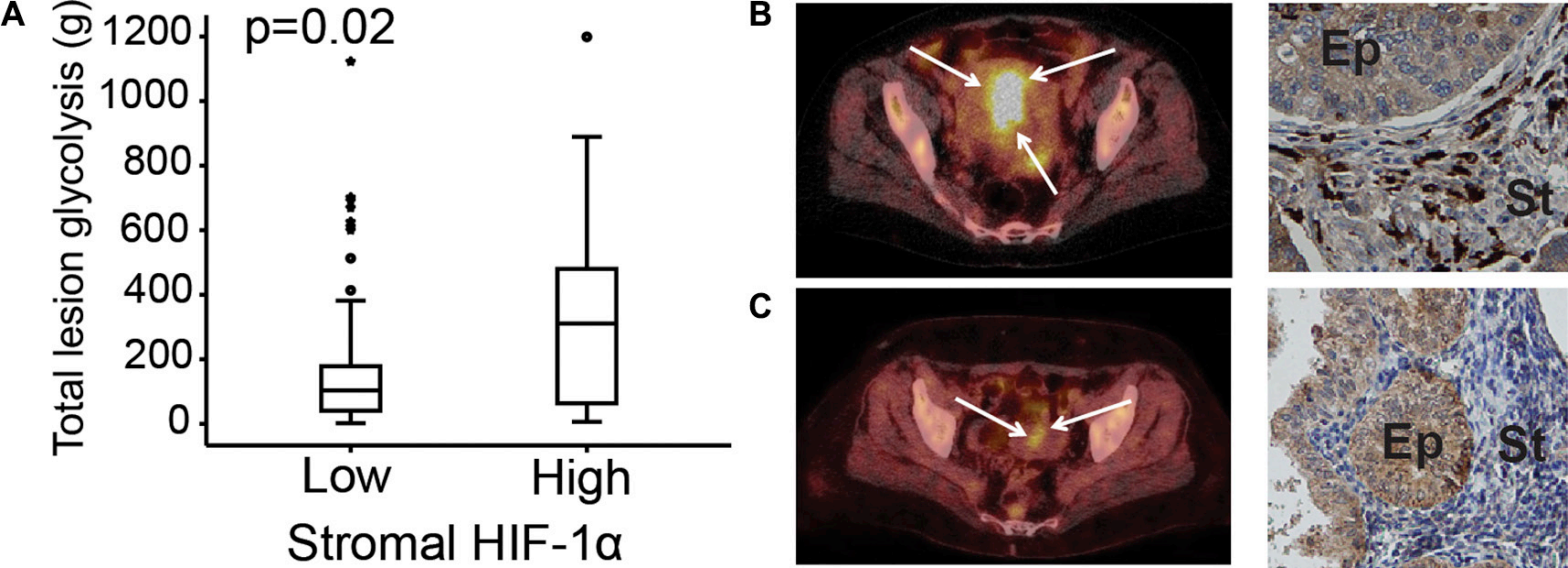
Stromal HIF-1α protein expression correlated to 18FDG PET/CT markers Total lesion glycolysis (TLG) assessed by FDG-PET/CT in primary endometrial carcinoma lesions compared to stromal HIF-1α protein expression (A). FDG-PET/CT and HIF-1α expression in a 77-year-old patient with a non-endometrioid endometrial cancer (NEEC), FIGO stage 1B: PET/CT exhibits a large FDG-avid uterine tumor (white arrows) with high TLG and the tumor had high stromal HIF-1α expression (black arrows) (B) FDG-PET/CT and HIF-1α expression in a 58-year-old patient with endometrioid endometrial cancer (EEC), grade 2, FIGO stage 1A: PET/CT exhibits a small uterine lesion (white arrows) with low TLG and the tumor had low stromal HIF-1α expression (black arrows) (C). Ep: epithelial tumor compartment. St: stromal tumor compartment.
